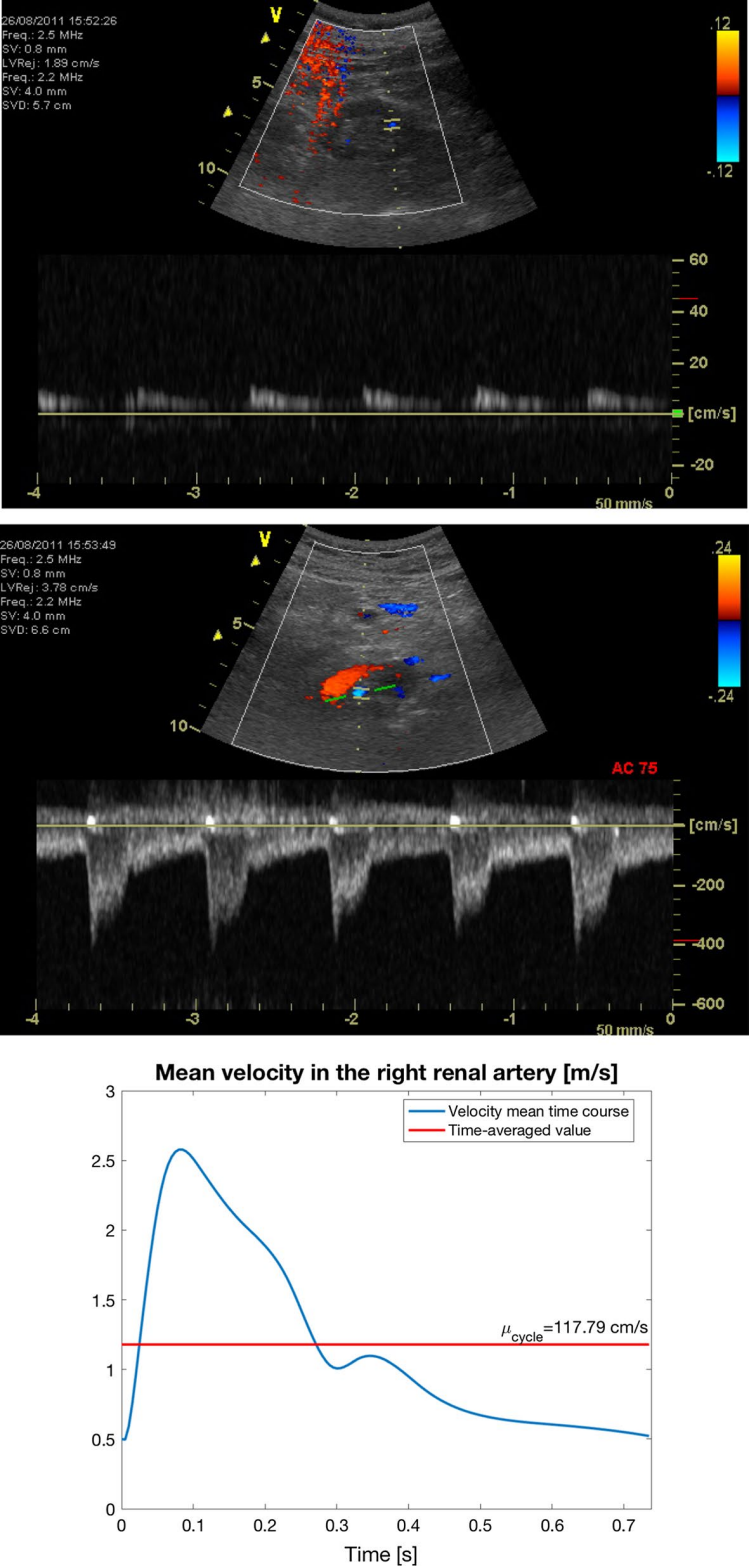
Doppler ultrasound images of the distal vessels in the left kidney (top) and in the right RA (in the middle) acquired for the same patient as the CT data shown in Fig. 2. Bottom: reconstruction of the mean velocity profile in the right RA from the DUS data within one cardiac cycle (blue line). The red horizontal line indicates the calculated velocity value averaged over the cycle duration
